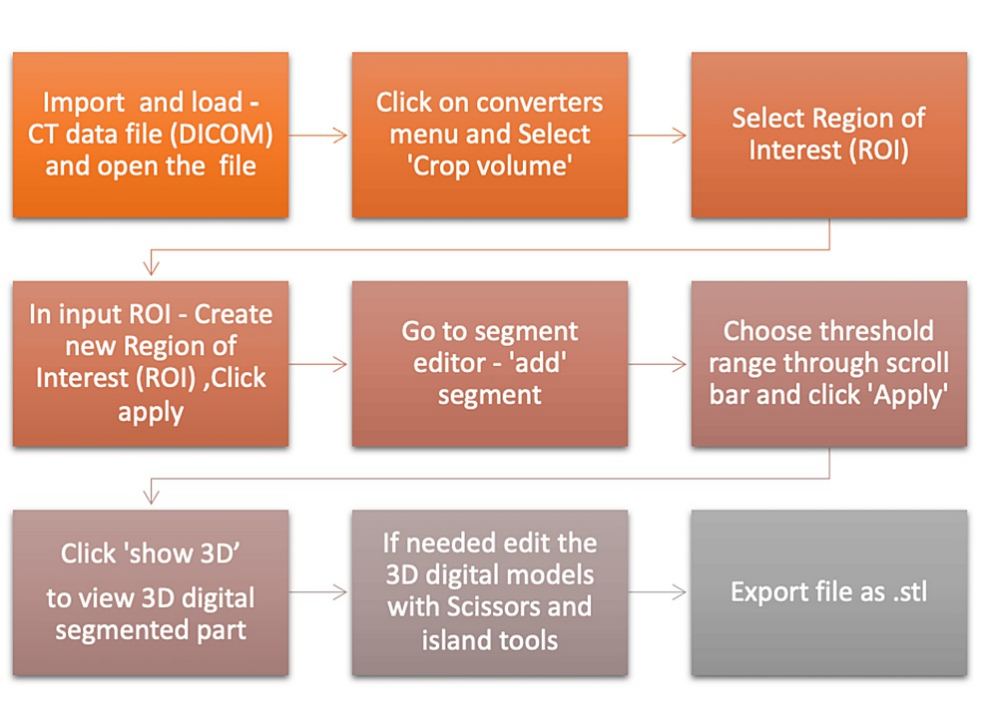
Process of image segmentation using 3D Slicer software from CT data Image created by Rathia DS and Konuri VK.
